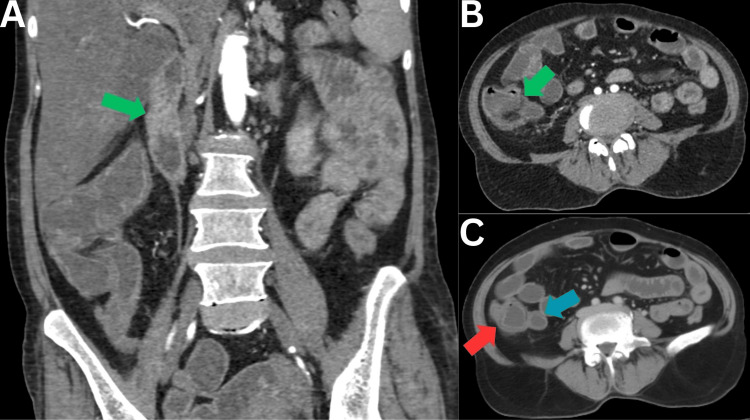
Contrast-enhanced CT coronal (A) and axial (B,C) sections of the abdomen and pelvis, showing edematous wall thickening involving the ileocecal junction (green arrows), cecum (red arrow), and the terminal ileum (blue arrow)
